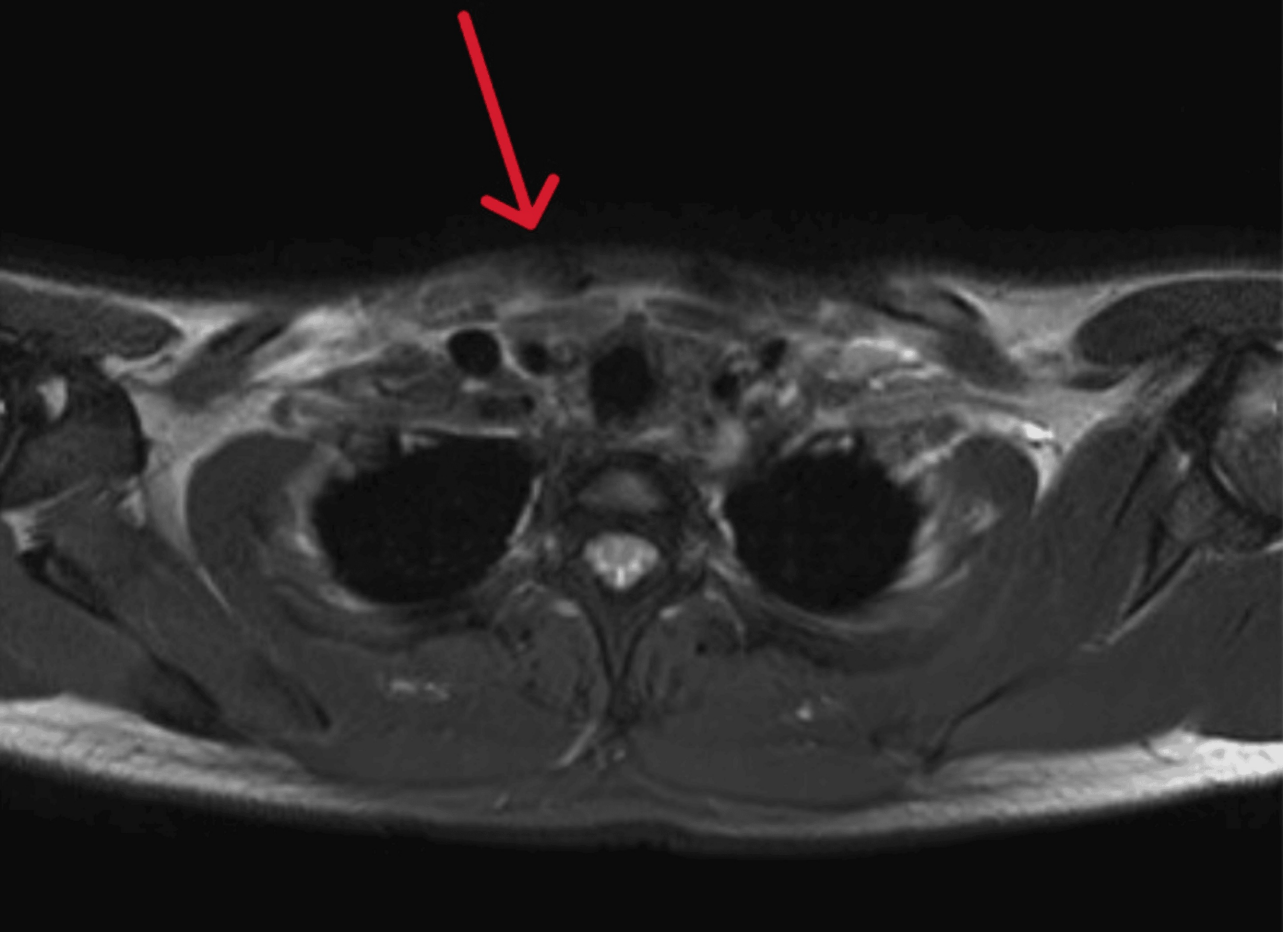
MRI of the chest (axial view) revealed soft tissue edema surrounding the proximal part of the clavicular head of the right sternocleidomastoid muscle (red arrow).
